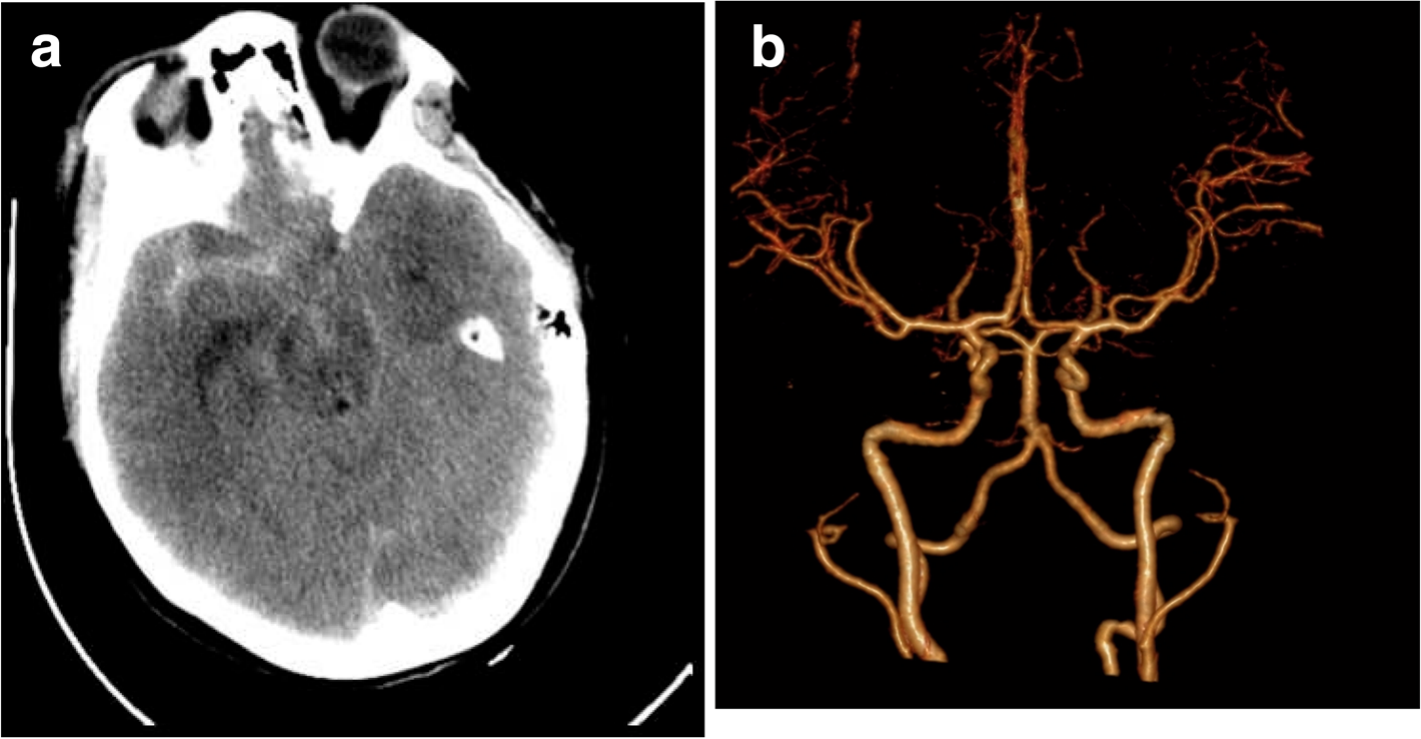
a Non-contrast CT head demonstrating the patient’s initial presenting scan with primarily right-sided subarachnoid hemorrhage. b CTA reconstructed images demonstrating normal bilateral vessel caliber
